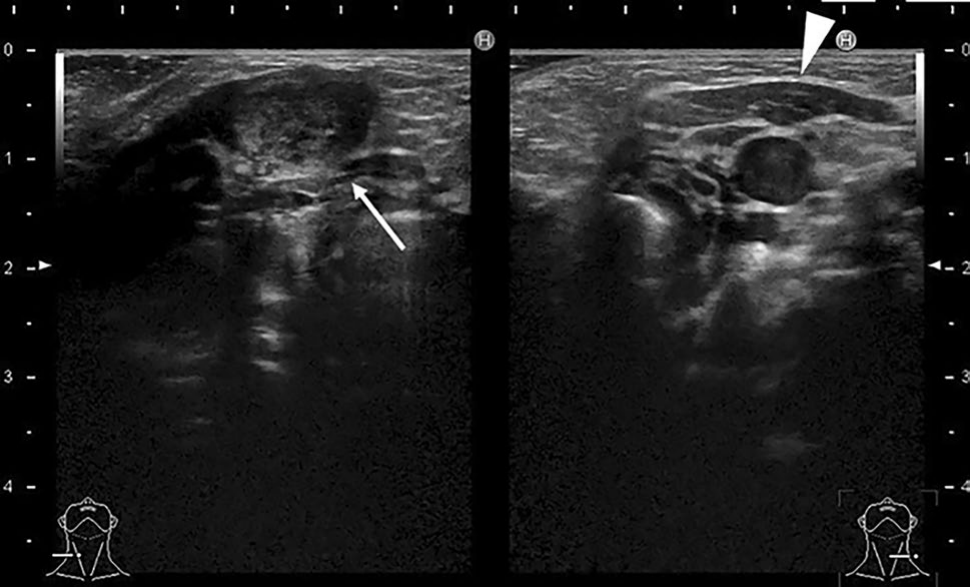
Two-month-old girl with hypothyroidism. Incidentally, fibromatosis colli of right sternocleidomastoid was detected. Ultrasonography revealed right sternocleidomastoid muscle (arrow) was thicker than that of left one (arrow head). Internal echogenicity of right sternocleidomastoid was high
